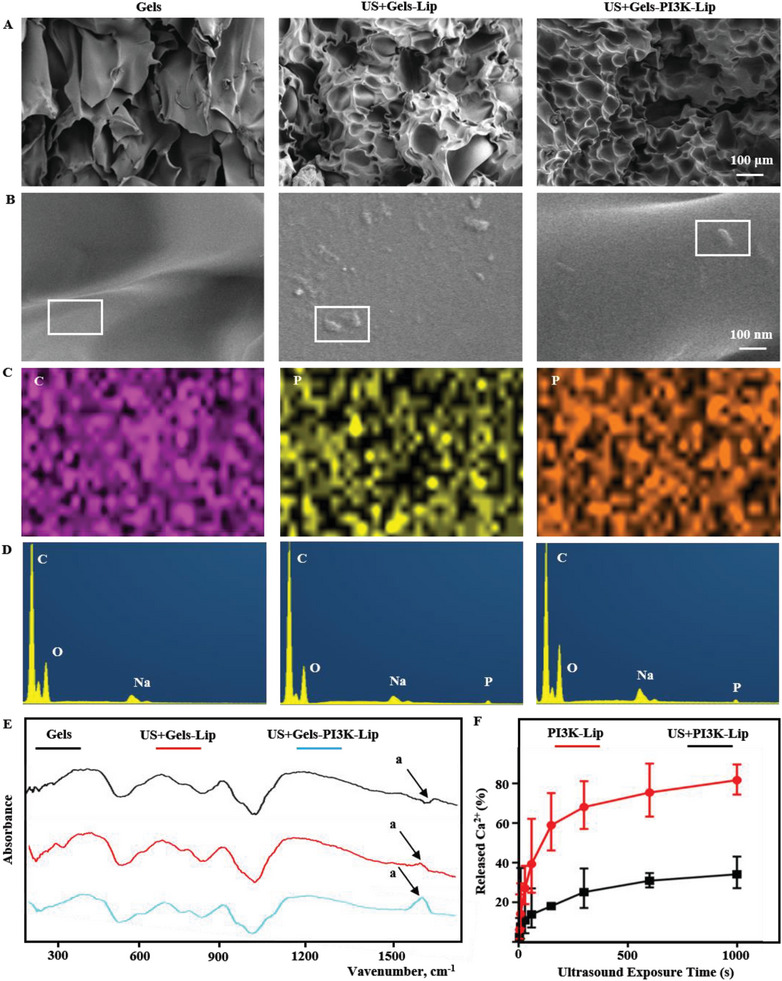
Related characterization of tissue‐penetrating ultrasound‐triggered hydrogel. A,B) SEM was used to detect the surface morphology of hydrogel. Scale bar: 100 µm and Scale bar: 100 nm. C,D) The distribution of related elements was detected in EDS mode. The grouping is consistent with parts A and B. E) FTIR detects changes in relevant chemical groups. F) The release of calcium ions after ultrasonic triggering.
